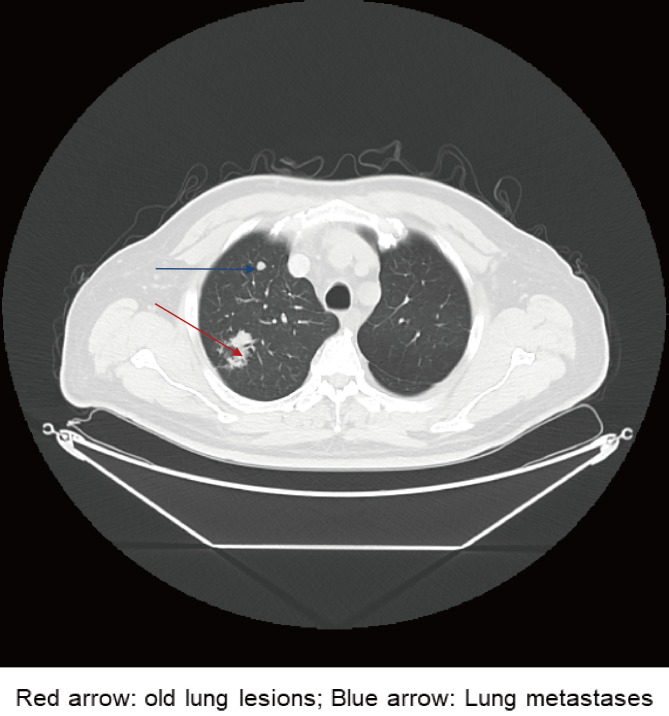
Post-neoadjuvant Chest CT. The blue arrow indicates a solitary nodule in the left lung, which was clinically suspected to be a pulmonary metastasis. The red arrow points to a benign, old pulmonary lesion (calcification/fibrosis) consistent with the patient's history of tuberculosis.
